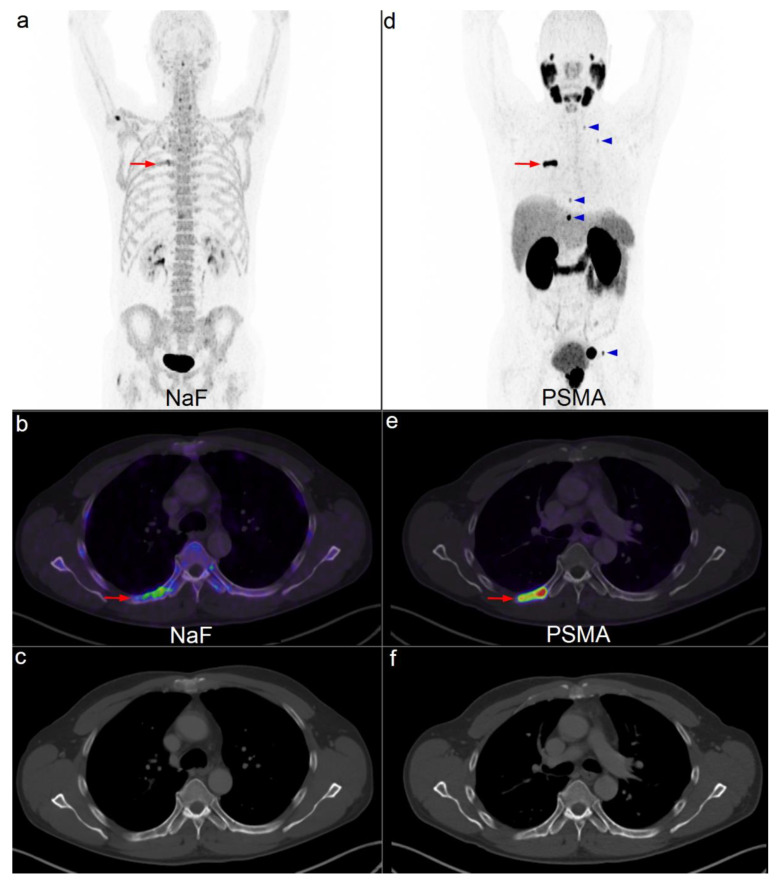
Example of a patient referred for ^18^F-NaF PET/low-dose CT for primary staging. An irregular lesion with faint uptake (*red arrow*) is visible on ^18^F-NaF PET/CT (a,b). Corresponding CT shows small, unspecific sclerotic areas (c). Subsequent ^68^Ga-PSMA PET/CT (d,e) shows intense uptake (*red arrow*). Several additional bone lesions with increased uptake are visible on maximum intensity projection (MIP) (d) (*blue arrowheads*). The corresponding CT is unchanged (f). PSMA MIP (d) also shows increased uptake in the prostate and in an enlarged lymph node in the left side of the pelvis.
